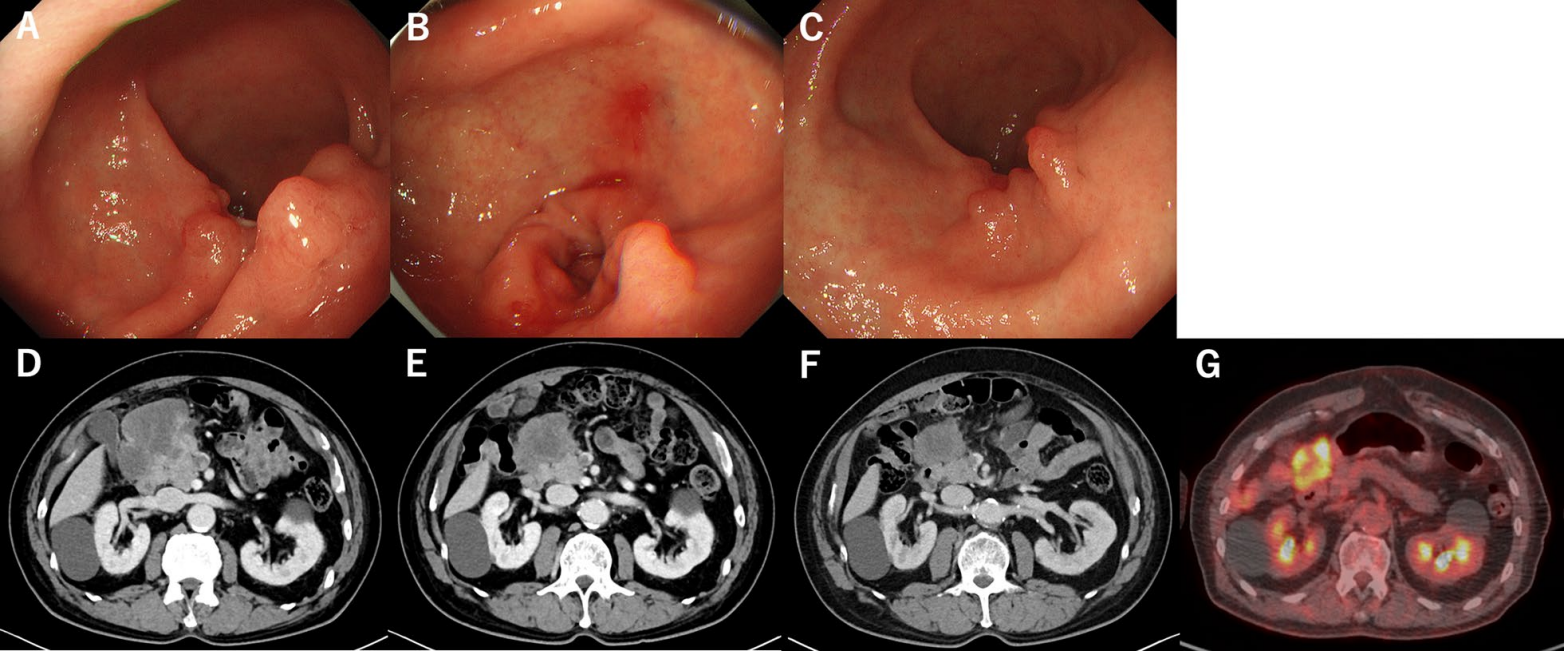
After three courses of SOX, the tumor had shrunk on esophagogastroduodenoscopy (A–C) and contrast-enhanced computed tomography scan (D–F). Positron emission tomography/computed tomography scan revealed that the FDG uptake in the tumor was decreasing (G)
